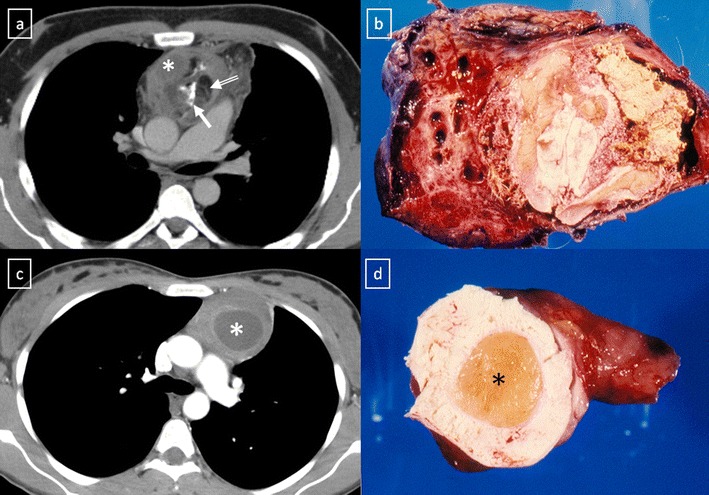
Chest imaging shows well the highly heterogeneous contents of mediastinal teratomas. a Mature cystic teratoma in a 40-year-old man. Contrast-enhanced CT scan shows a heterogeneous anterior mediastinal mass with areas of fat (*open arrow*), calcification (*arrow*) and fluid attenuation (*). Posterior displacement of mediastinal structures is also be seen*.*
